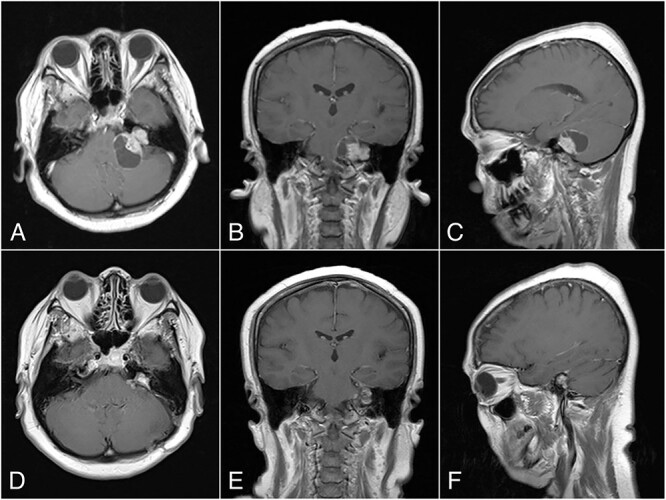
Preoperative MRI, (A) Axial, (B) Coronal, and (C) Sagittal plane, shows a “dumbbell” shaped vestibular schwannoma. Postoperative MRI, (D) Axial, (E) Coronal, and (F) Sagittal plane, shows near total resection with some residual mass on the left CPA.
